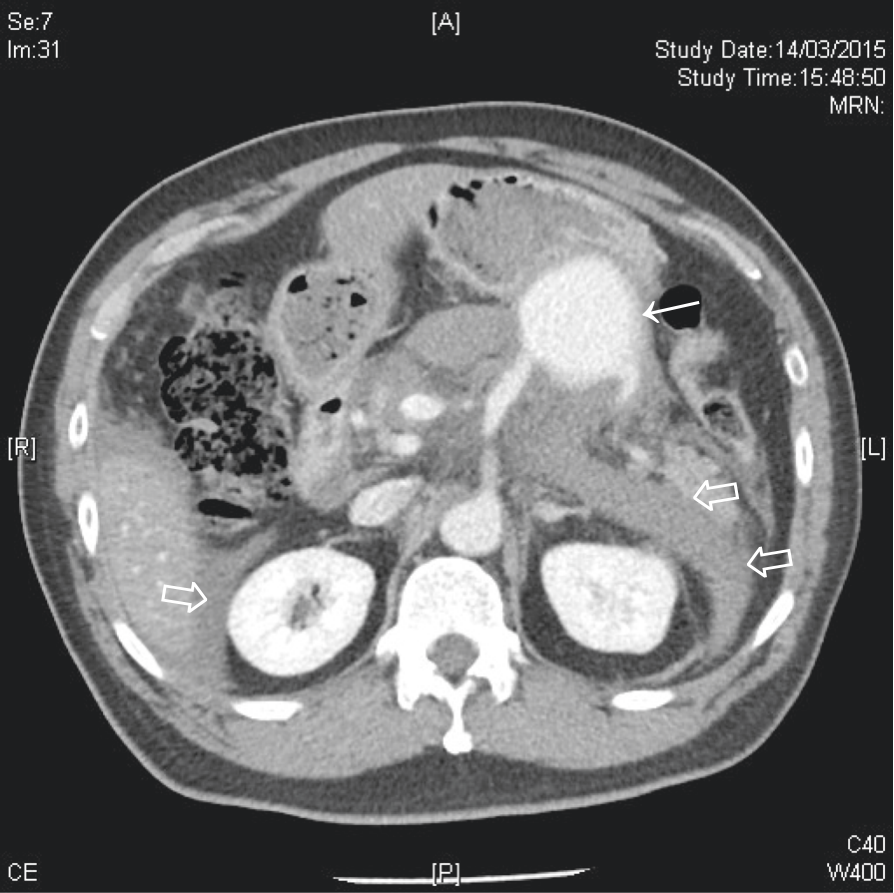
CT scan of the abdomen with IV contrast showing a contrast-enhanced mass [←] at the splenic artery (branching from the superior mesenteric artery) compatible with a splenic artery pseudoaneurysm. Hemoretroperitoneum and hemoperitoneum were demonstrated [⇦]
